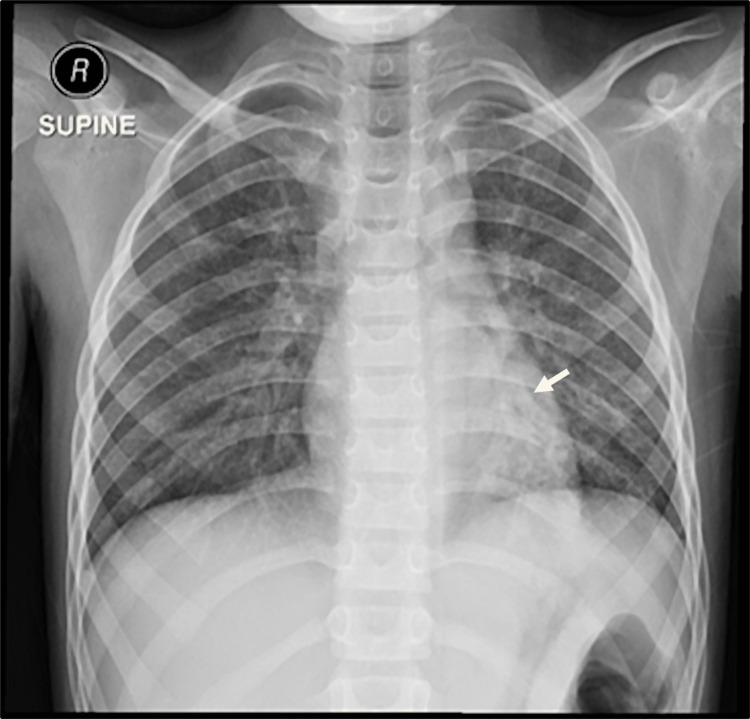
Chest X-ray The chest X-ray evaluation showed airspace opacity over the left lower lobe.
